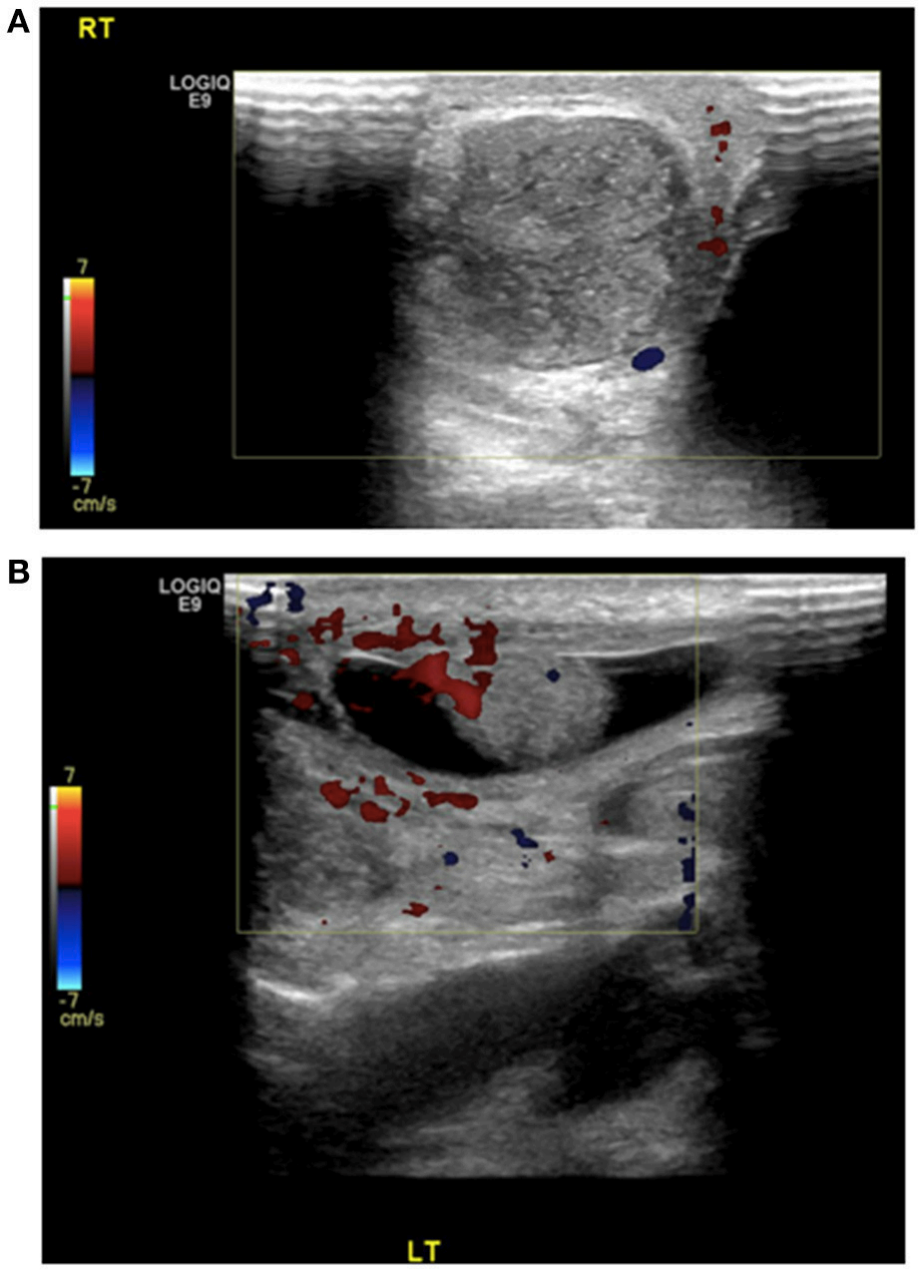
Doppler US showed (A) no blood flow to the right testis with (B) preservation of the vascularity of the left testis.
